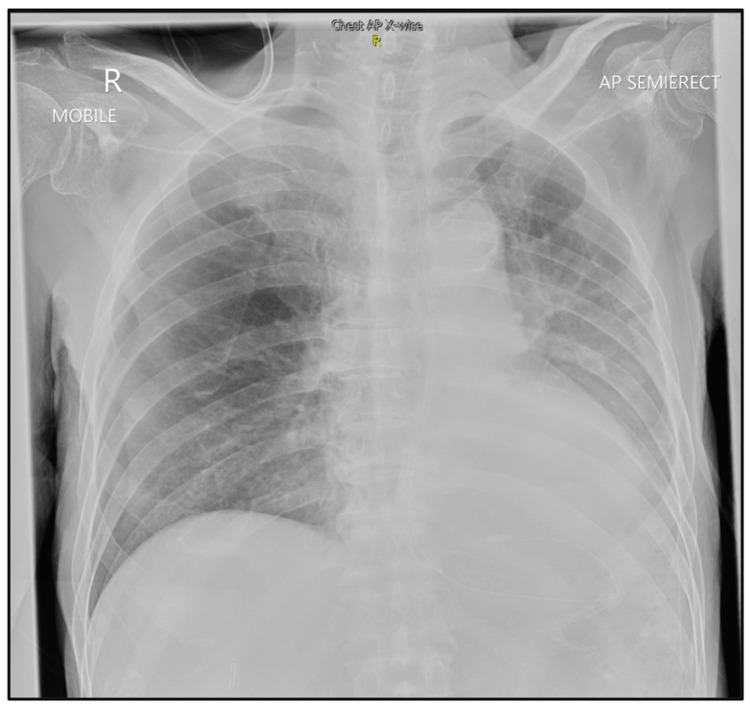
Chest radiograph obtained on day 4 of admission. AP, anteroposterior.
